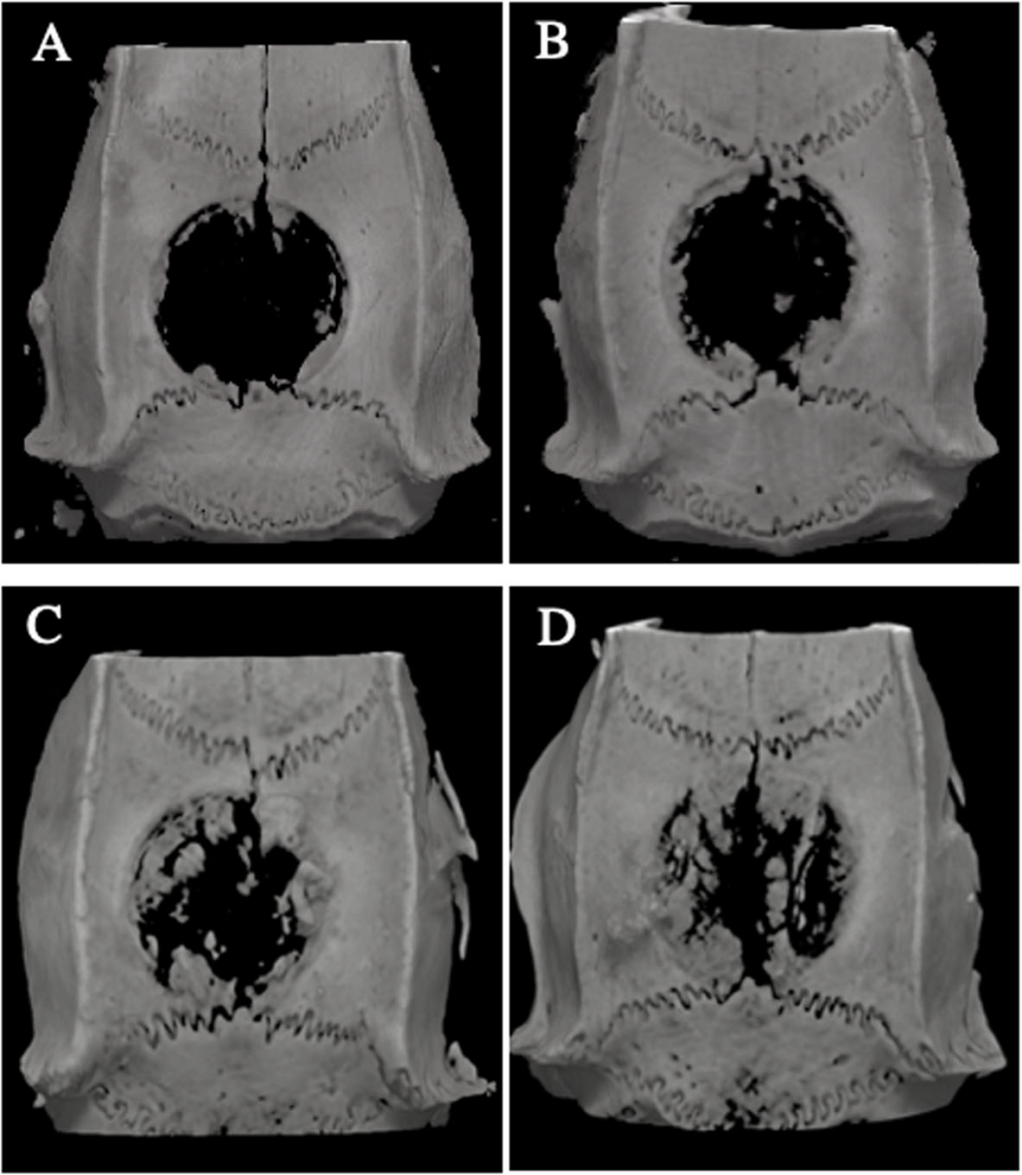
Radiographic analysis was performed. a 3D images at 1 week in the control. b 3D images at 1 week in the test. c 3D images at 2 weeks in the control. d 3D images at 2 weeks in the test
